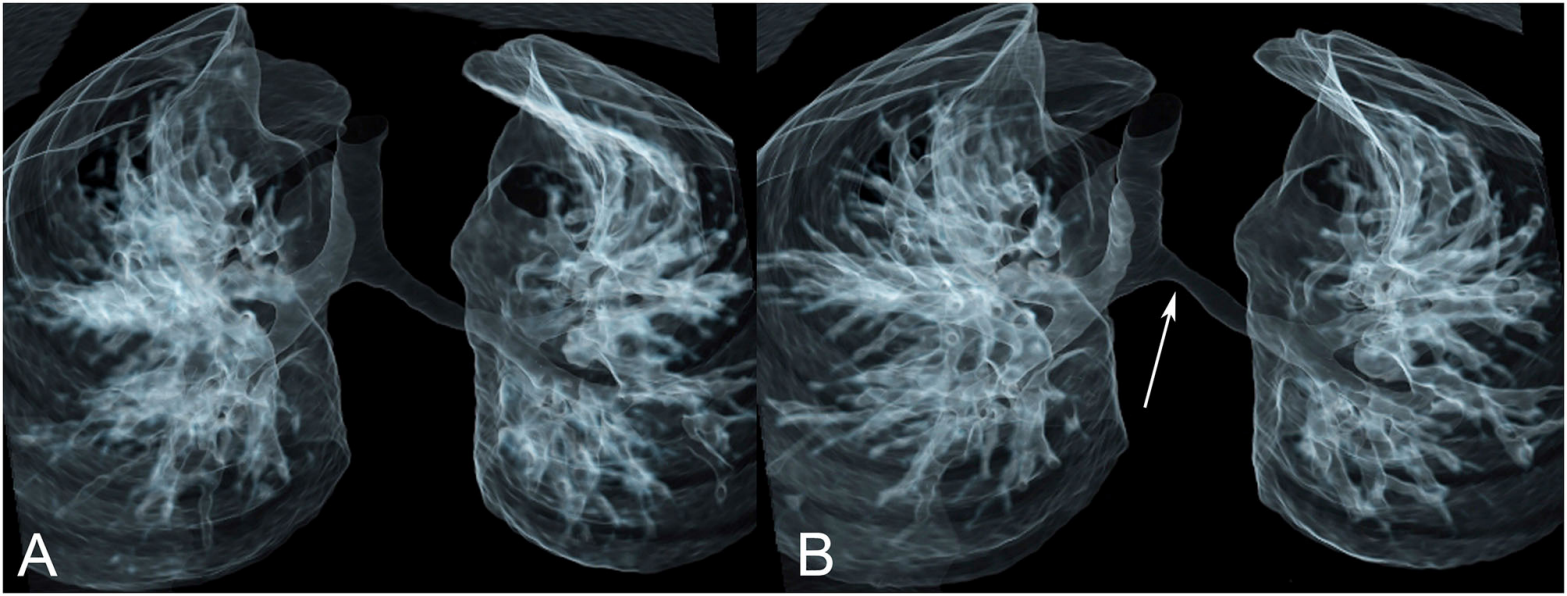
Tracheobronchomalacia. Paired images from a dynamic (“4D”) CT study in inspiration (A) and expiration (B) show focal collapse of the left main bronchus in expiration (arrow).
